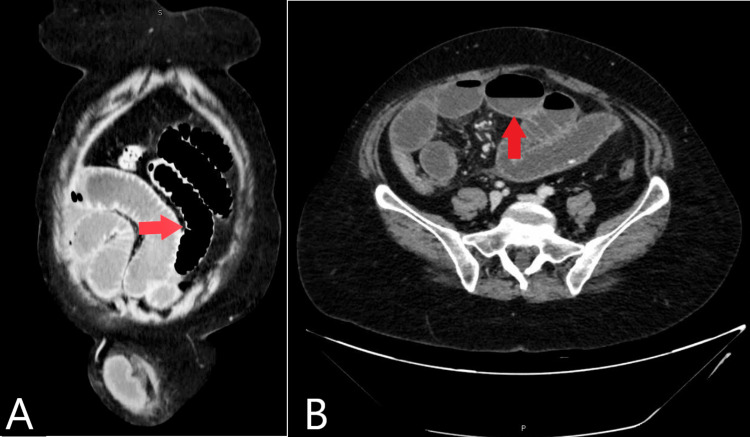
CT of the abdomen and pelvis (A) Coronal view with red arrow to demonstrate small bowel obstruction up to proximal jejunum. (B) Sagittal view with red arrow to demonstrate multiple air-fluid levels suggest small bowel obstruction.
